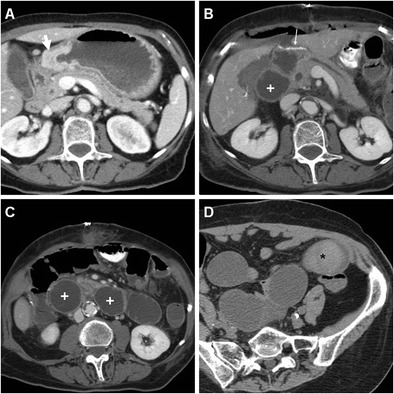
A 72-year-old female with distal gastric carcinoma (thick arrow) as preoperatively depicted by CT (A) underwent total gastrectomy. On 6th POD the patient suffered from persistent abdominal pain and anemization. Postoperative CT (B-D) showed dilated fluid-filled duodenum (+) up to stapled DS, consistent with afferent limb syndrome, secondary to a large blood clot in the jejunum which was best seen as a hyperattenuating intraluminal mass (*) on precontrast (D) scans. Surgical reintervention was required to relieve obstruction
